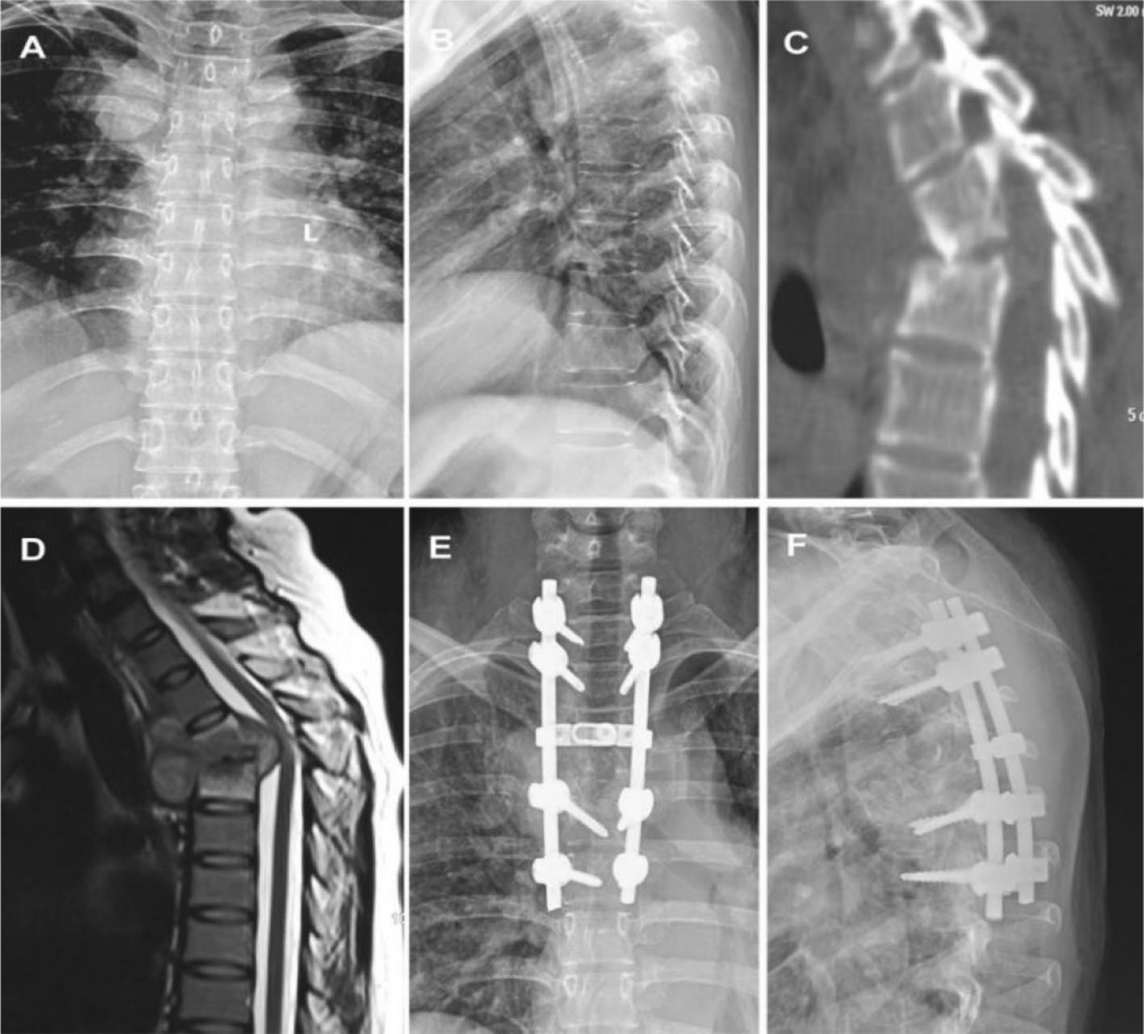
The patient (female; 43 years old) presented with thoracic tuberculosis at T3-4 and underwent single posterior debridement, bone graft fusion and instrumentation. (A, B): X-ray in the positive and lateral position before surgery; (C, D): preoperative CT and MRI examination; (E, F): x-ray in the positive and lateral position after surgery.
